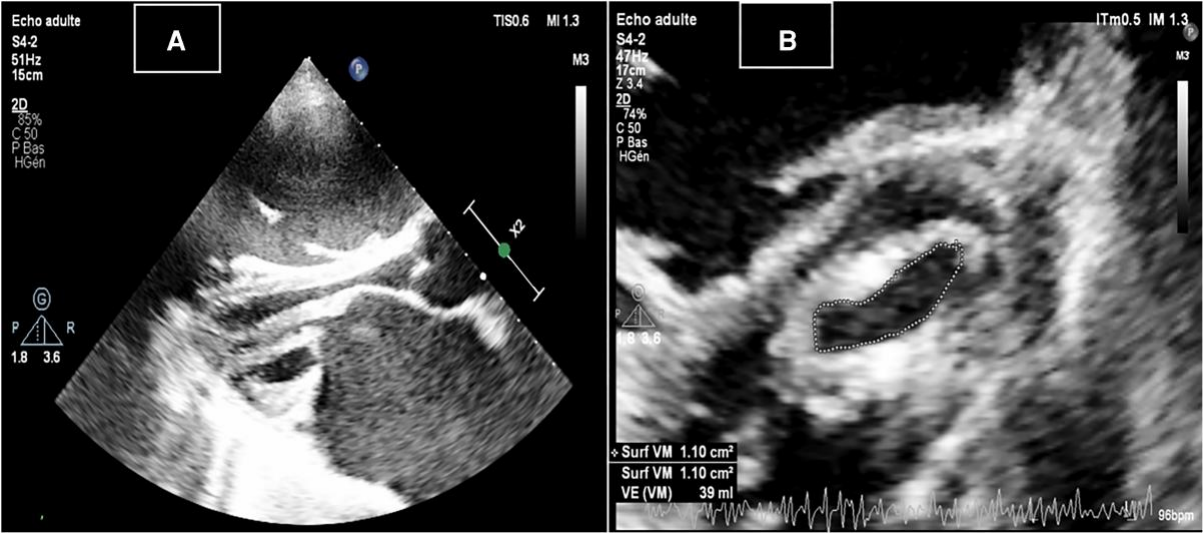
(*A*) Transthoracic echocardiogram parasternal long-axis view revealing thickened mitral leaflets with restricted opening in diastole. (*B*) Transthoracic echocardiogram parasternal short-axis of the mitral valve showing severe mitral stenosis with a mitral area of 1.1 cm^2^.
